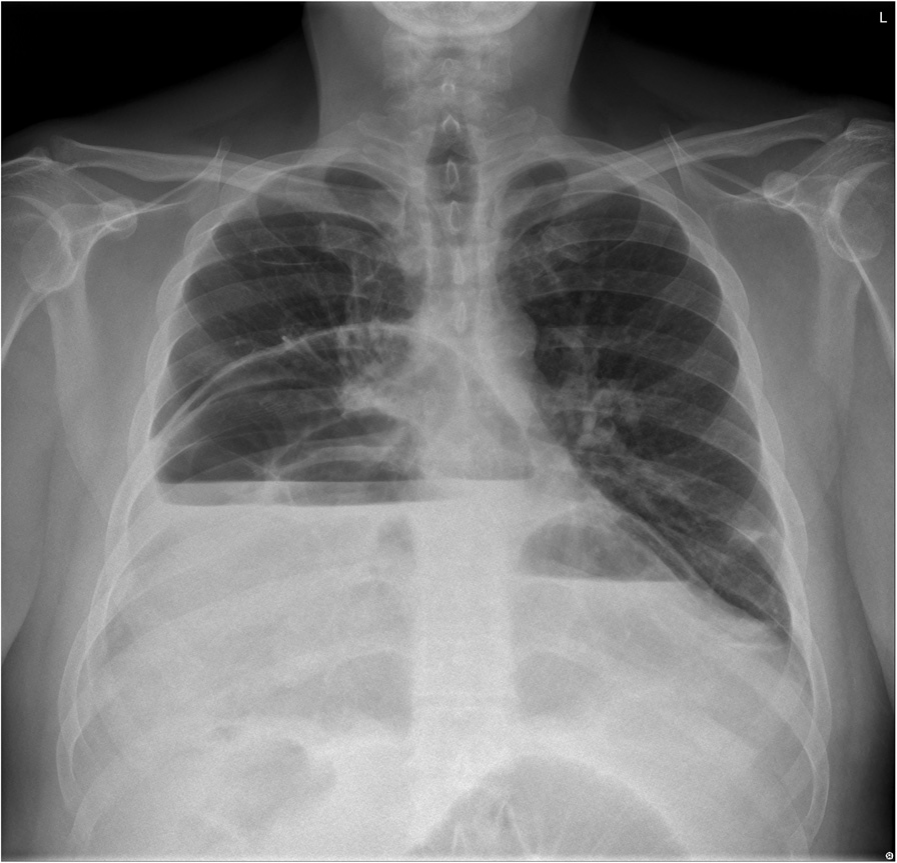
Preoperative X-ray. X-ray of the chest revealed air-fluid levels on right and left hemithorax.
